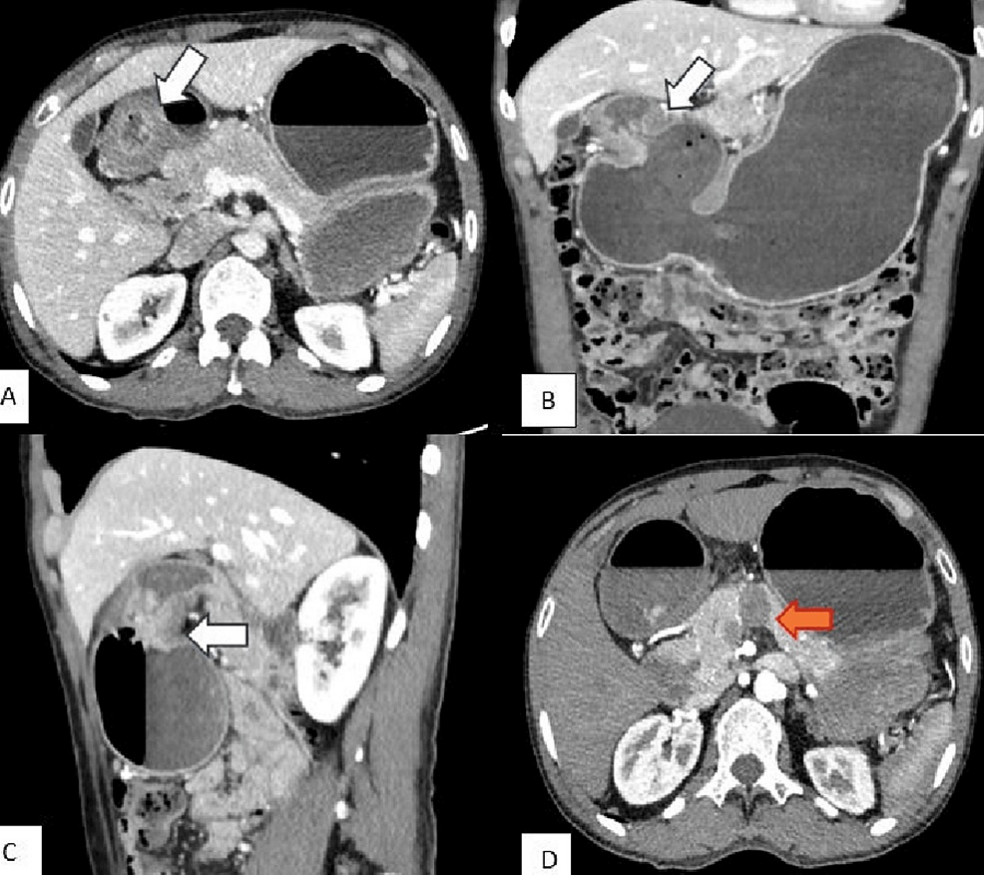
Computed tomography (CT) scan of the abdomen (A-axial, B-coronal, C-sagittal views): showing diffuse dilatation of the stomach up to the antropyloric level with abrupt narrowing in the pyloric region (white arrow) over a length of approximately 2.4 cm caused by circumferential and asymmetric wall thickening. D – Axial view: showing a large hypodense peri-pancreatic lymph node measuring 22 mm x 17 mm (red arrow).
